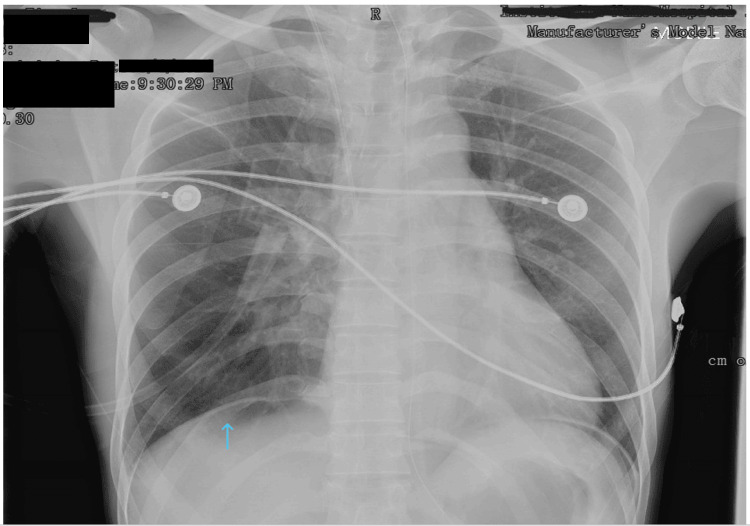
Abdominal X-ray showing air under diaphragm (blue arrow)
